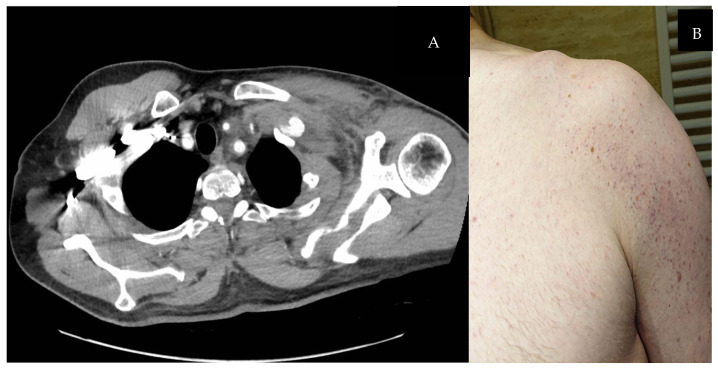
(A) Chest CT scan (axial plane): pseudoaneurysm misinterpreted as a massive hematoma of the supraclavicular region. (B) Patient’s subclavian region and shoulder during admitting.
